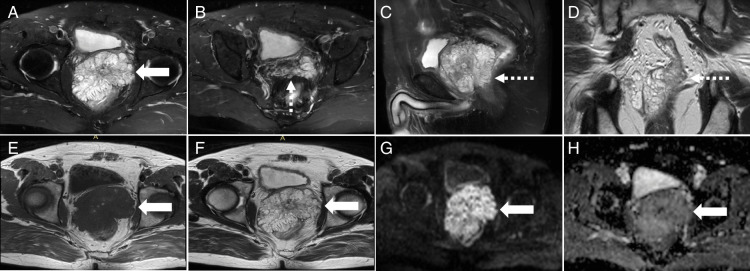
A 62-year-old man presented with progressive dysuria for 3 years without fever, hematuria, or abdominal pain. MRI revealed a multi-cystic mass in the prostatic region, measuring 9.2 × 6.3 × 8.1 cm (A, white arrow). Axial (B) and sagittal (C) fat-suppressed T2-weighted imaging (T2WI) and coronal T2WI (D) demonstrated a multi-septate lesion invading the seminal vesicles and anterior rectal wall (dashed arrows). The cystic fluid (white arrows) showed mixed hypo- and hyper-intensity on axial T1WI (E) and T2WI (F). Diffusion-weighted imaging (DWI) revealed hyper-intensity (G), while the apparent diffusion coefficient (ADC) map showed hypo-intensity (H), indicating restricted diffusion within the cystic fluid. These imaging features strongly suggested prostate mucinous adenocarcinoma.
